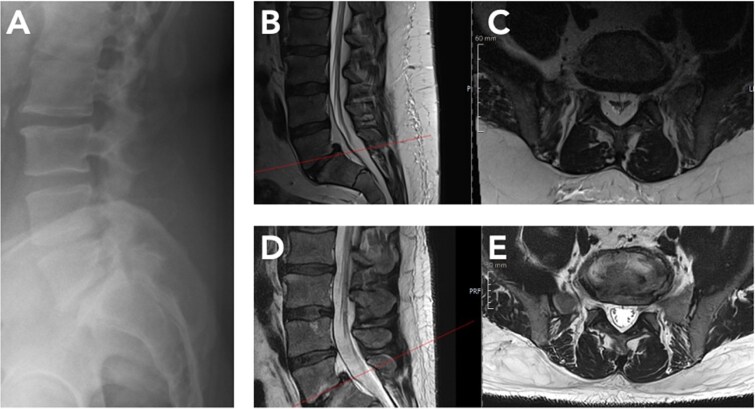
Radiological regression of lumbar spinal epidural lipomatosis (L5/S1) between October 2024 and September 2025. (A) Lateral lumbar spine radiograph (October 2024) showing preserved vertebral alignment without instability but indirect signs of reduced dorsal epidural space at L5/S1. (B, C) Baseline MRI (October 2024). Sagittal and corresponding axial T2-weighted sequence at L5/S1 level demonstrates marked epidural fat accumulation at L5/S1 with compression of the thecal sac. (D, E) Follow-up MRI (September 2025) after massive GLP-1–induced weight loss. Sagittal and corresponding axial T2-weighted image at L5/S1 level shows substantial reduction of epidural fat with re-expansion of the thecal sac.
